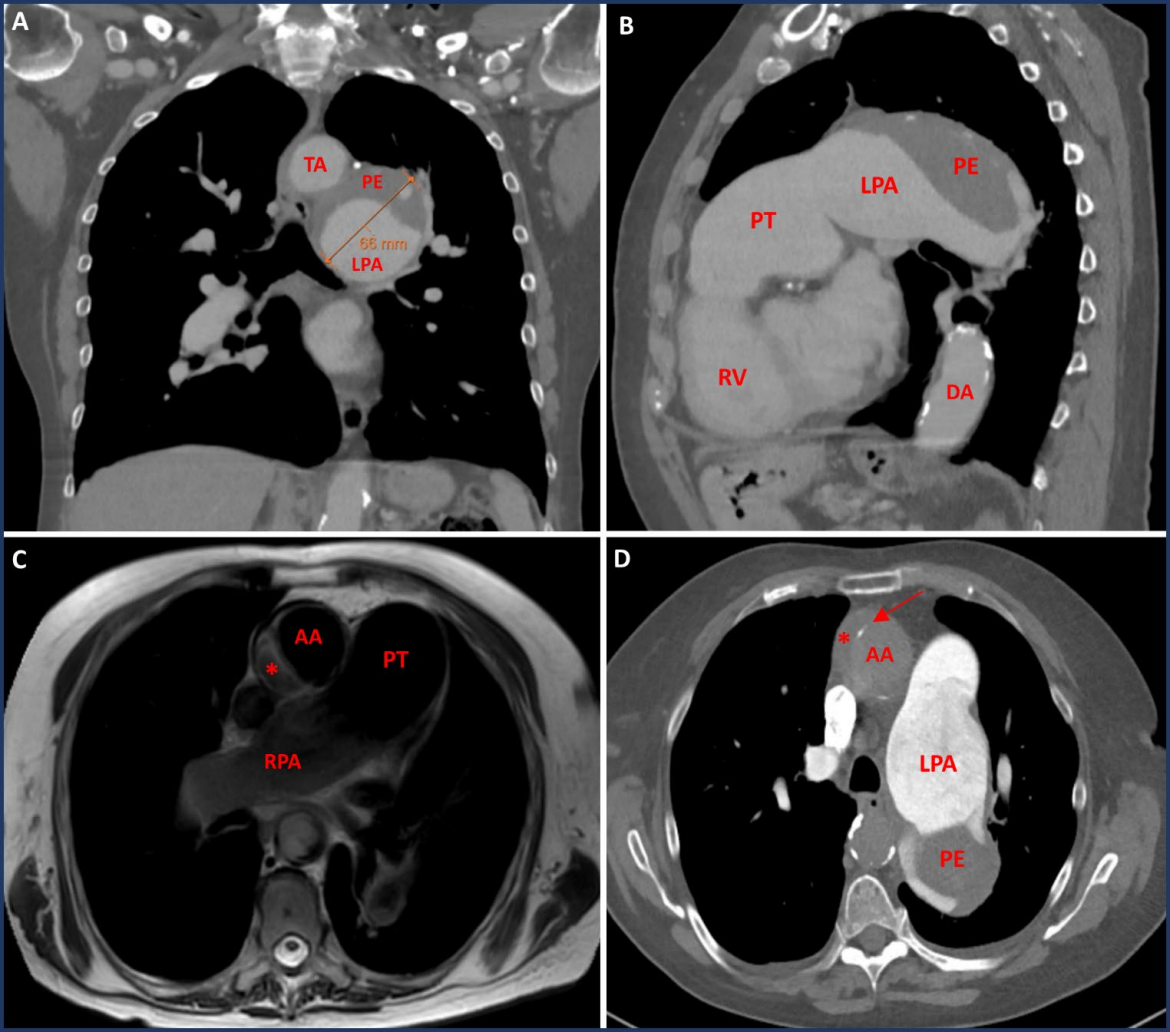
Diagnostic work-up imaging with CMR and CT scan of the PAA and PE. Initial CT and CMR scan in the diagnostic work-up regarding the PAA and PE. Figure 1A and B: CT scan with frontal and sagittal view of the transverse aortic arch and left pulmonary artery with pulmonary embolism. Figure 1C and D: CMR and CT scan with transversal view of the aneurysmatic pulmonary trunk, right and left pulmonary artery, as well as the ascending aorta with retrospectively diagnosed saccular post-dissection aneurysm (depicted by *). The arrow indicates calcifications marking the aortic lumen AA = ascending aorta, DA = descending aorta, LPA = left pulmonary artery, PE = pulmonary embolism, PT = pulmonary trunk, RPA = right pulmonary artery, RV = right ventricle, TA = transverse aortic arch
